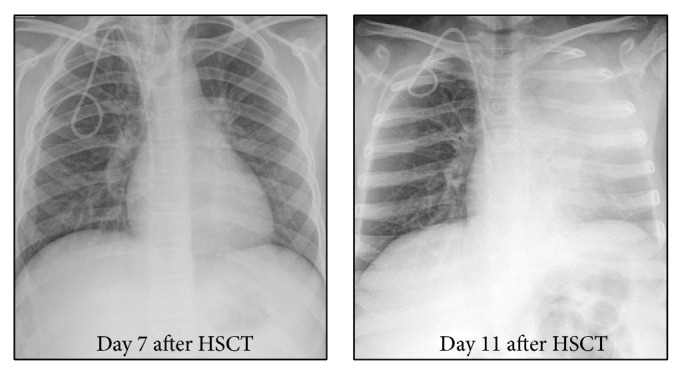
Chest X-rays performed on days 7 and 11 following transplant. No lung abnormality is seen at day 7 but left upper lobe collapse has developed by day 11 after HSCT.
